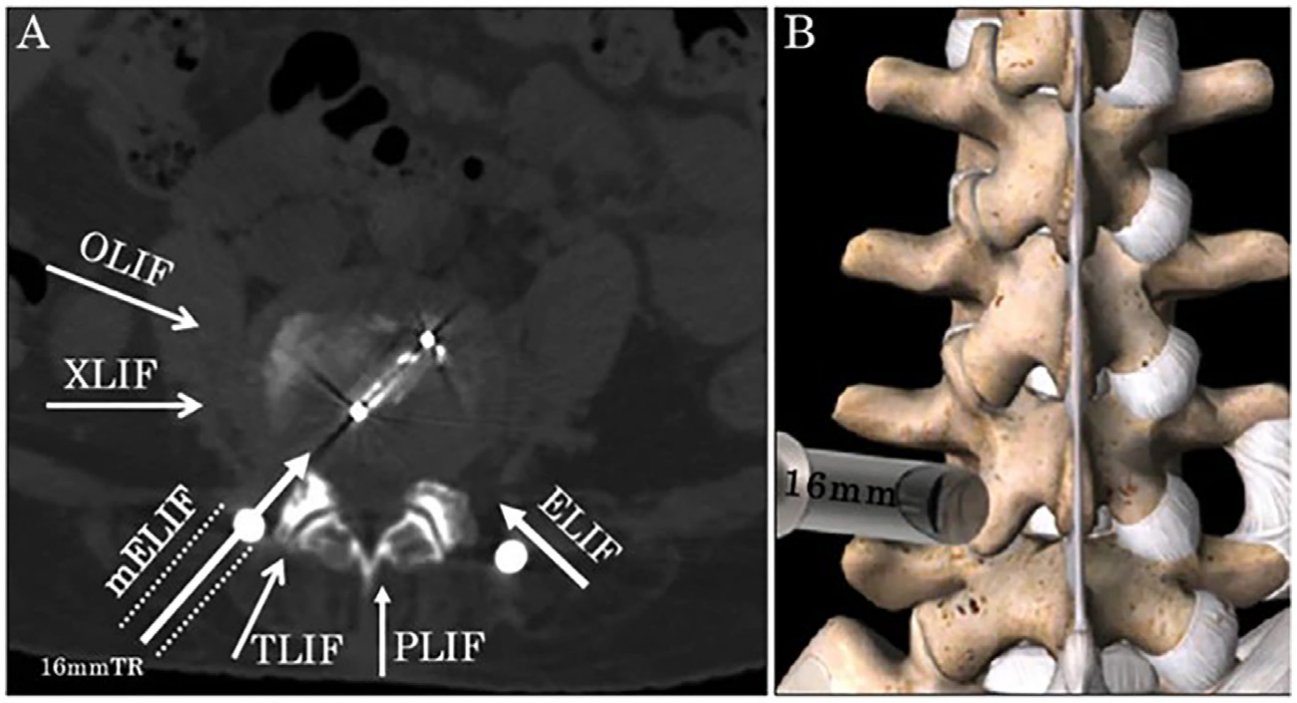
Representative postoperative images after mELIF (AP and lateral radiographs; sagittal and axial MRI; CT demonstrating fusion around the cage) (Fig. 4) [17].
